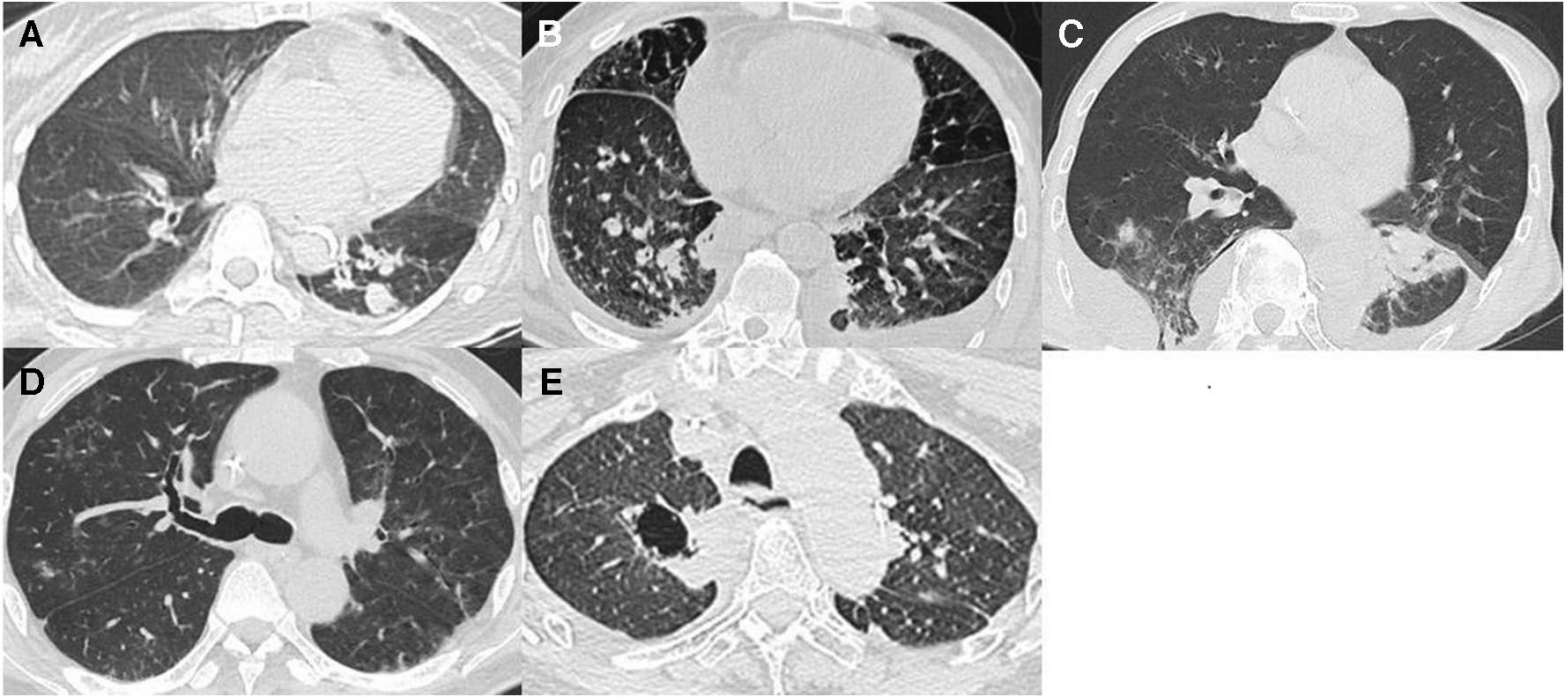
Representative examples of chest CT findings in IA patients. A Aspergillus nodules in the right upper and lower lobes and left lower lobe. B An Aspergillus nodule with pleural effusion in the left lobe. C Consolidation in the right middle lobe and left upper lobe. D Bronchiectasis in the right lower and middle lobes. E An Aspergillus nodule with cavitary lesions in the right middle lobe.
